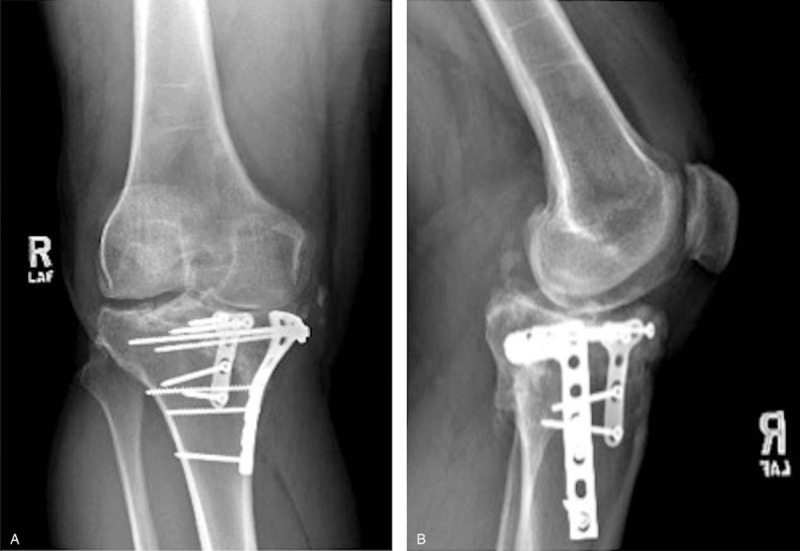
1A: Preoperative AP x-ray demonstrating retained hardware from previous tibial plateau ORIF performed in 2009. 1B: Lateral x-ray of Figure 1A.
